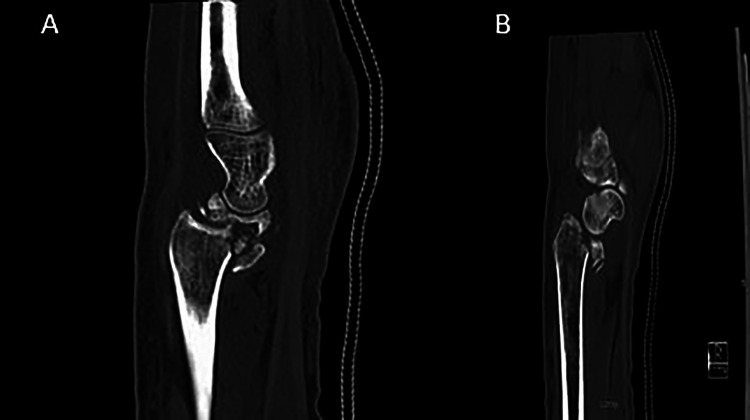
(A and B) Sagittal slices of the computed tomography image outlining the incongruity of the scaphoid fossa. Figure 1A also outlines the collapse of the lunate.
